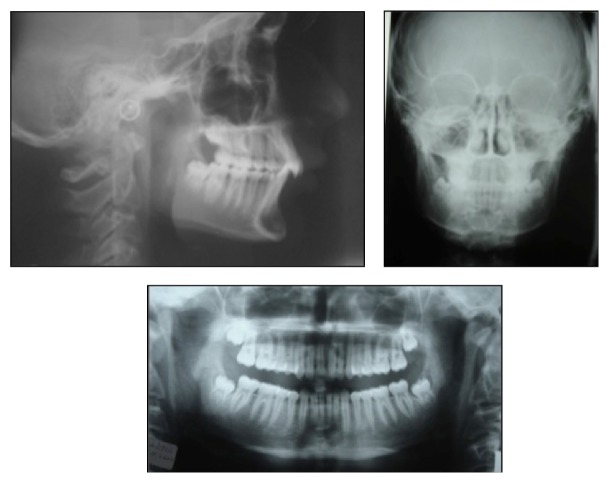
Pretreatment lateral, frontal, and panoramic radiographs.
